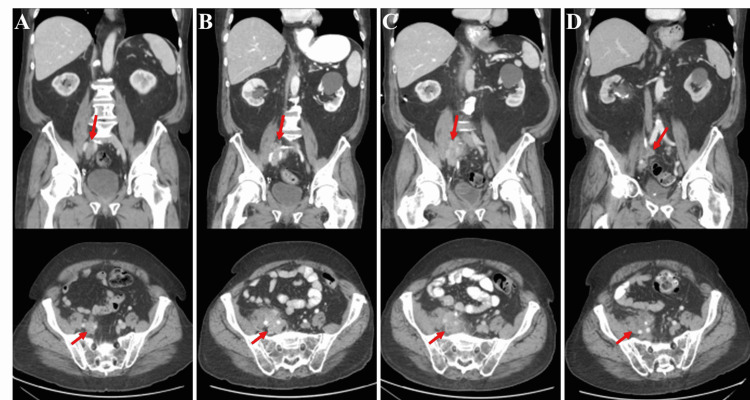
Serial CT of the abdomen and pelvis demonstrating interval changes in tumor burden in December 2016, January 2018, May 2018, and September 2019 (left to right) (A) Ill-defined lobular soft tissue mass (1.7 x 1.3 cm) below the bifurcation of the right common iliac vessel. (B) Interval increase in soft tissue mass size, measuring 4.3 x 3.5 cm. (C) Slightly reduced soft tissue mass size (4.1 x 3.5 cm). (D) Interval increase in the size of lobulated soft tissue mass (4.9 x 3.7 cm), now encasing distal right ureter CT: computed tomography
